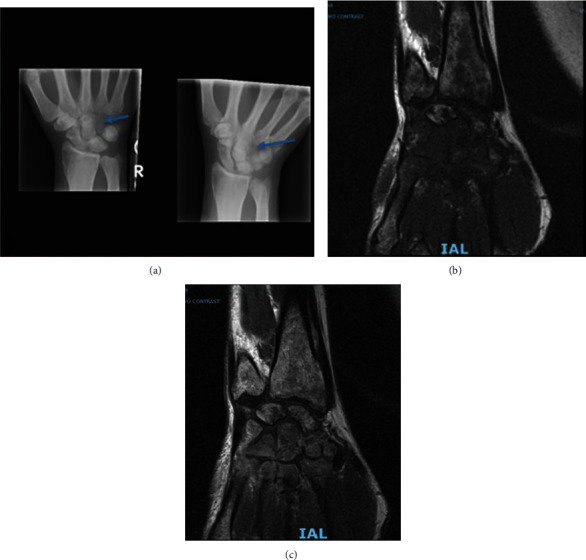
(a) Plain film X-ray of the right hand reveals diffuse severe lucency of the entire hamate bone (blue arrows) with a thin peripheral cortex visible. (b) T1 MRI. (c) T2-weighted MRI with contrast of the right hand reveals severe bone marrow replacement throughout the distal radius and ulna, carpal bones, and metacarpals with complete replacement of the trapezoid, capitate, and hamate.
